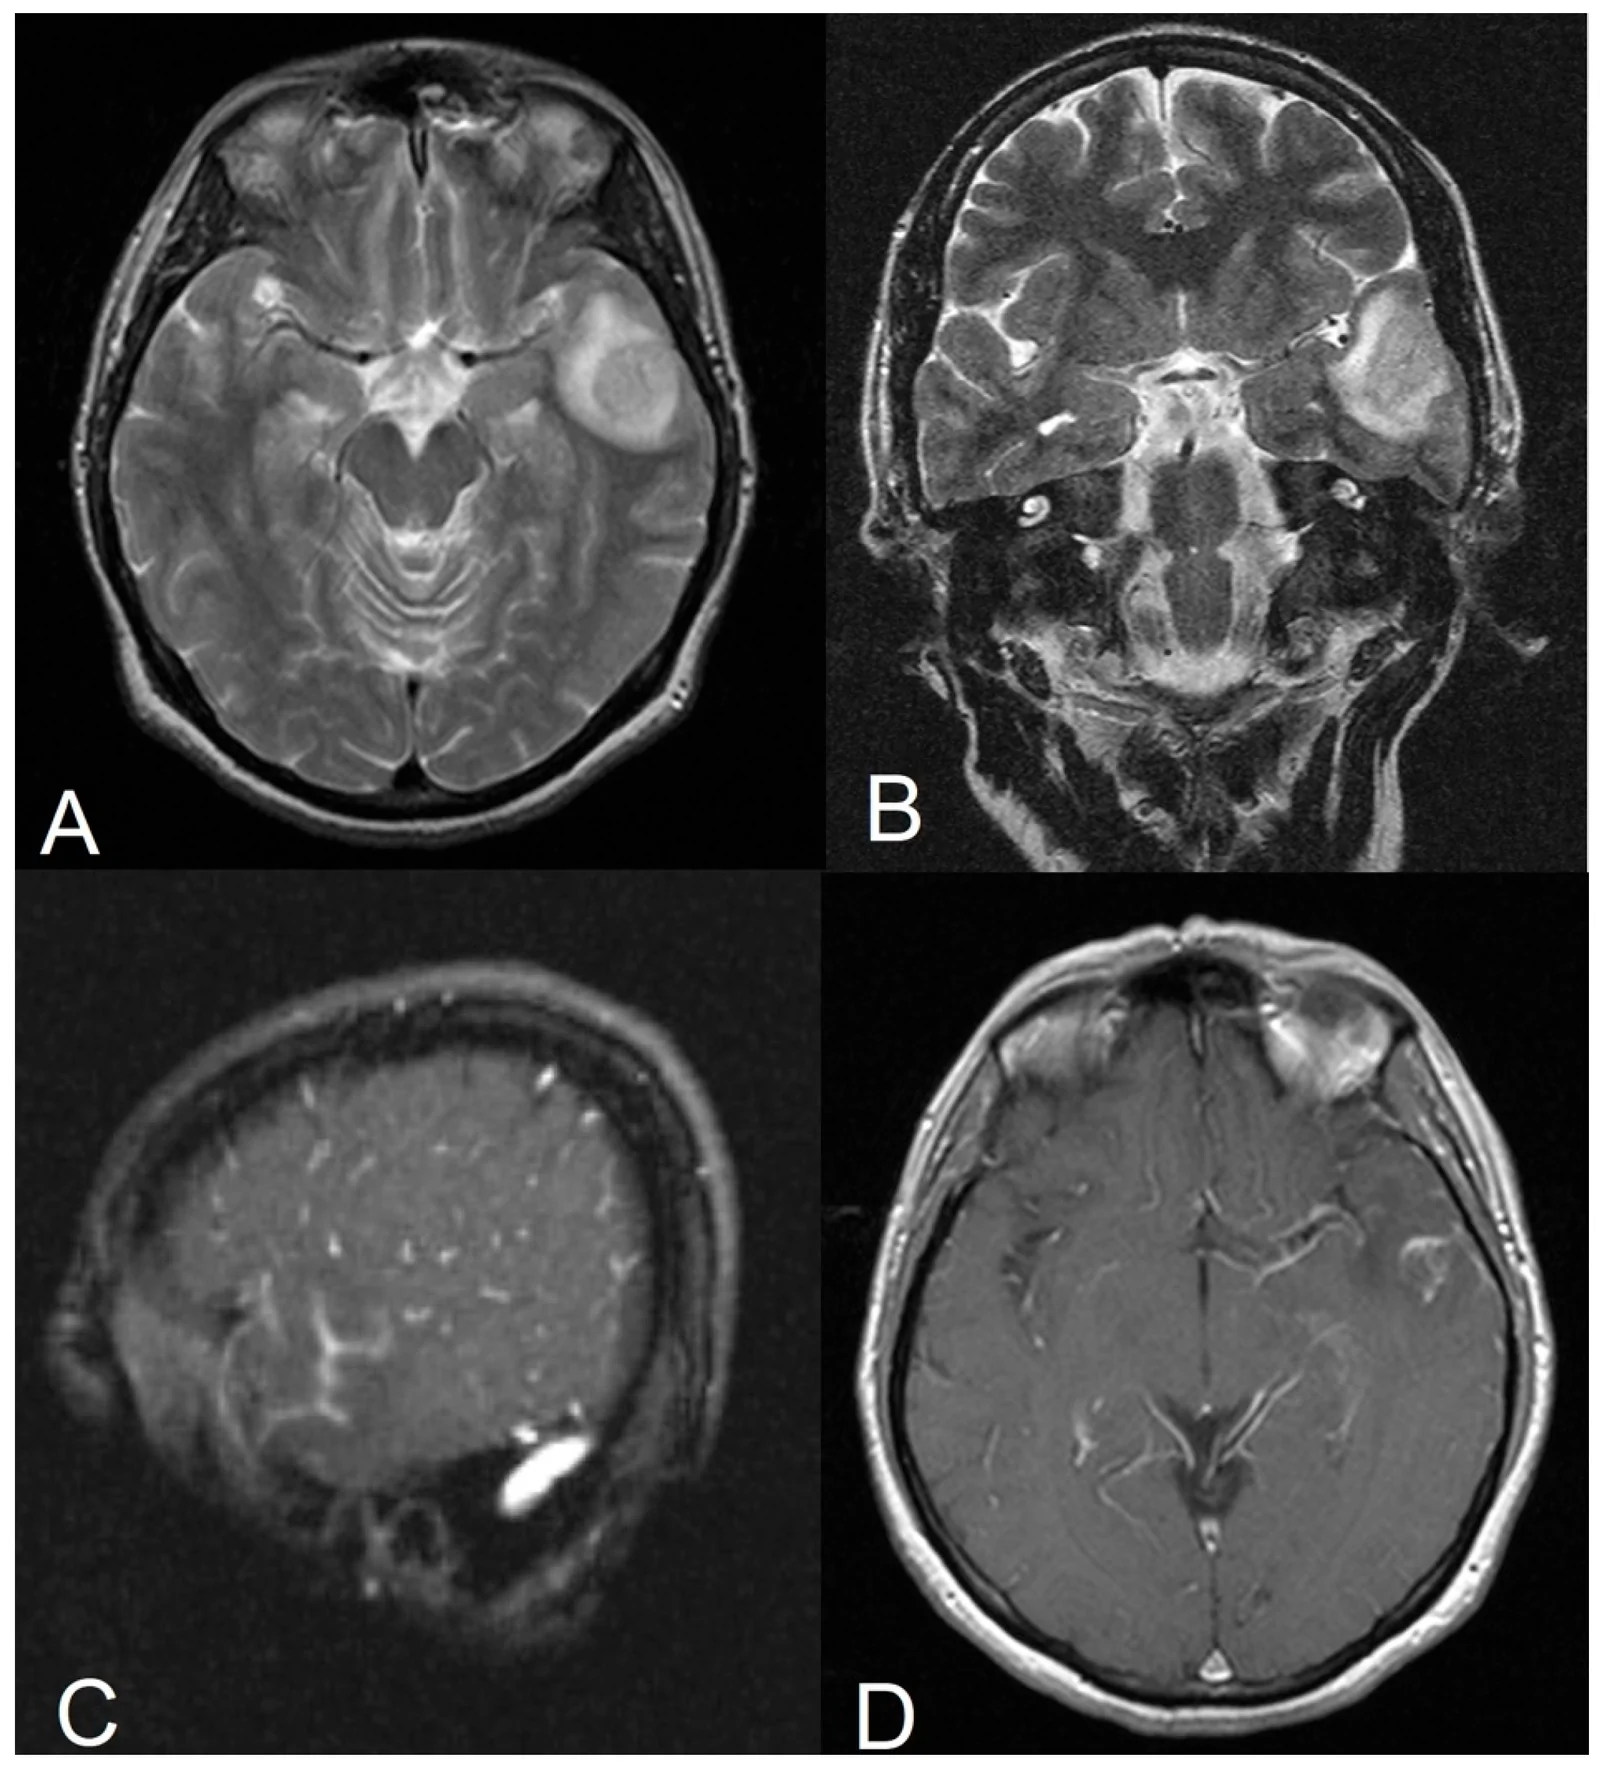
(A) Axial and (B) coronal T2-weighted MR images presenting abnormal hyperintensity within the anterior aspect of the left temporal lobe. Notable changes include focal gyri thickening, effacement of the adjacent sulci, blurring of the subcortical gray–white matter junction and concomitant predominantly vasogenic edema. (C) Sagittal and (D) axial T1-weighted post-contrast images revealing prominent focal leptomeningeal enhancement in the left anterior temporal region and adjacent lateral frontal operculum. The radiographic differential diagnosis primarily includes focal encephalitis versus a low-grade glial neoplasm.
